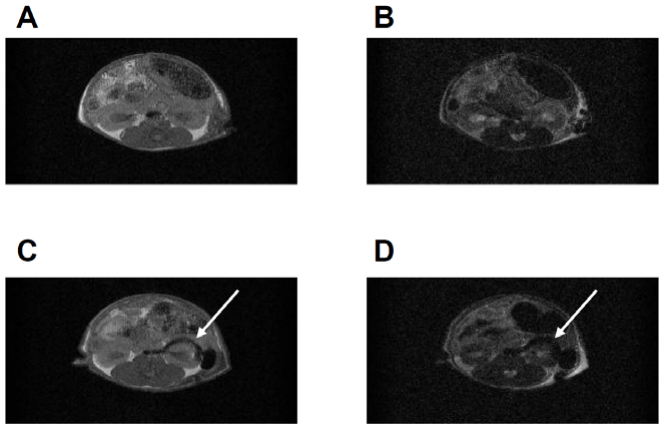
In vivo MRI of transplanted MIN6 cells labeled with TMADM-03. A. T1-weighted images of MIN6 cells without TMADM-03 transplanted into the left kidney capsule. B. T2-weighted images of MIN6 cells without TMADM-03 transplanted into the left kidney capsule. C. T1-weighted images of MIN6 cells labeled with TMADM-03 transplanted into the left kidney capsule. D. T2-weighted images of MIN6 cells labeled with TMADM-03 transplanted into the left kidney capsule. The arrows show the transplanted graft.
